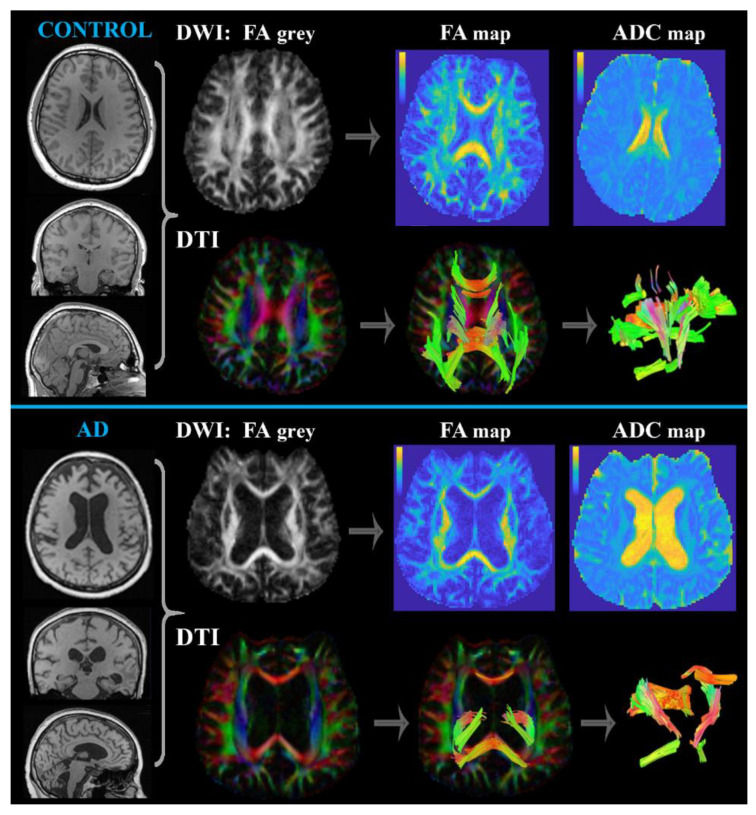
Example of MRI (T_1_-weighted MRI at 3 Tesla MR-scanner), DWI, and DTI (performed on DSI studio software incorporating MATLAB postscripts) neuroimaging of a control subject (50-year-old male) and AD patient (56-year-old female). AD exhibits more restricted diffusion in the tissue, a worse global network density, and lost white matter fiber tracts. Abbreviations: ADC, apparent diffusion coefficient; DTI, diffusion tensor imaging; DWI, diffusion weighted imaging; FA, fractional anisotropy.
